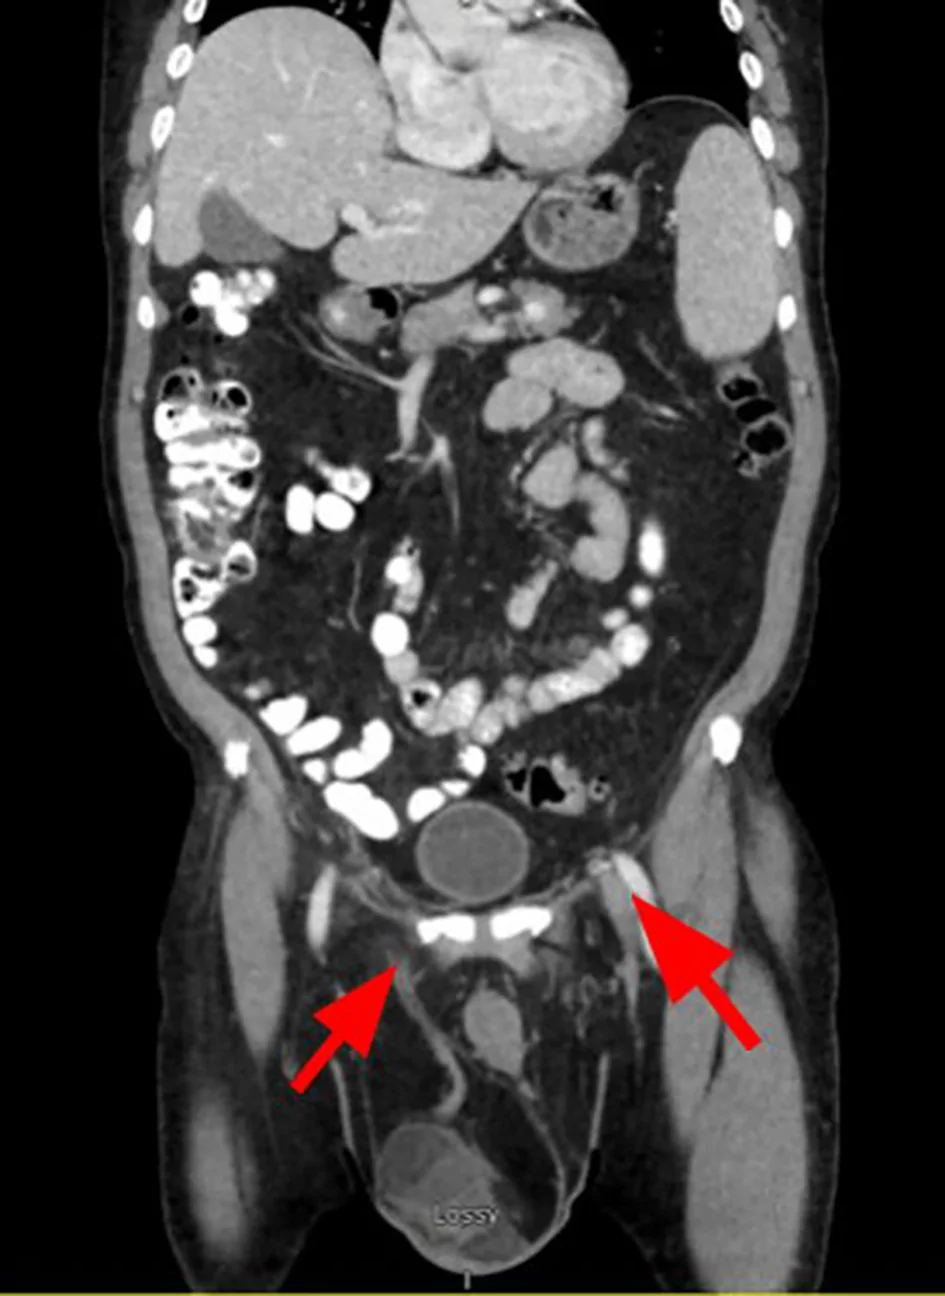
Coronal contrast-enhanced CT of the abdomen and pelvis demonstrating bilateral fat-containing inguinal masses (red arrows). The larger right-sided lesion extends through the inguinal canal into the right hemiscrotum and was initially interpreted as an inguinal hernia containing mesenteric fat. Retrospective review demonstrated internal soft tissue nodularity and mass effect concerning liposarcoma. CT: computed tomography.
